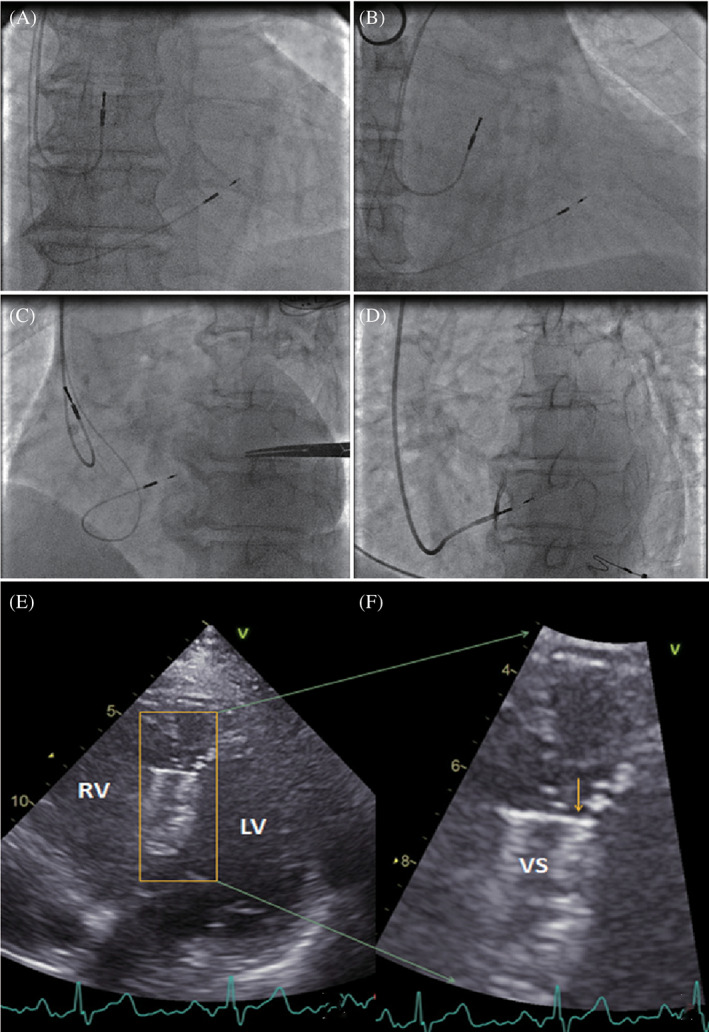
LBBP lead location confirmed by fluoroscopy and post‐implant 2D echocardiography. Fluoroscopic imaging when the pacing lead was placed in the left bundle branch area which was beneath the left side of the ventricular septum in orthotopic view, the right anterior oblique 30° view and the left anterior oblique 40° view, A‐C. Contrast injection through the sheath in the left anterior oblique 35° view, D. F, Enlarged picture of, E, to show the pacing lead tip under the left endocardium of the septum. The arrow indicates the location of the pacing lead tip with a high density. LBBP, left bundle branch pacing; LV, left ventrium; RV, right ventrium; VS, ventricular septum
